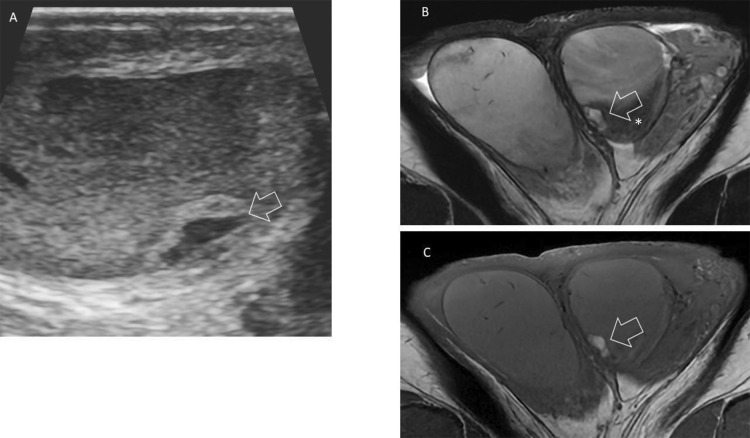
(A) Follow up ultrasound showed a lesion size reduction with newly appearing anechoic component on(arrow). T2 (B) and T1‑weighted images (C) showed a newly appearing hypersignal of the lesion compatible with the presence of extracellular methemoglobin (arrow) and newly hypointense area on the T2‑weighted image surrounding the lesion corresponding to segmental testicular infarction (*).
